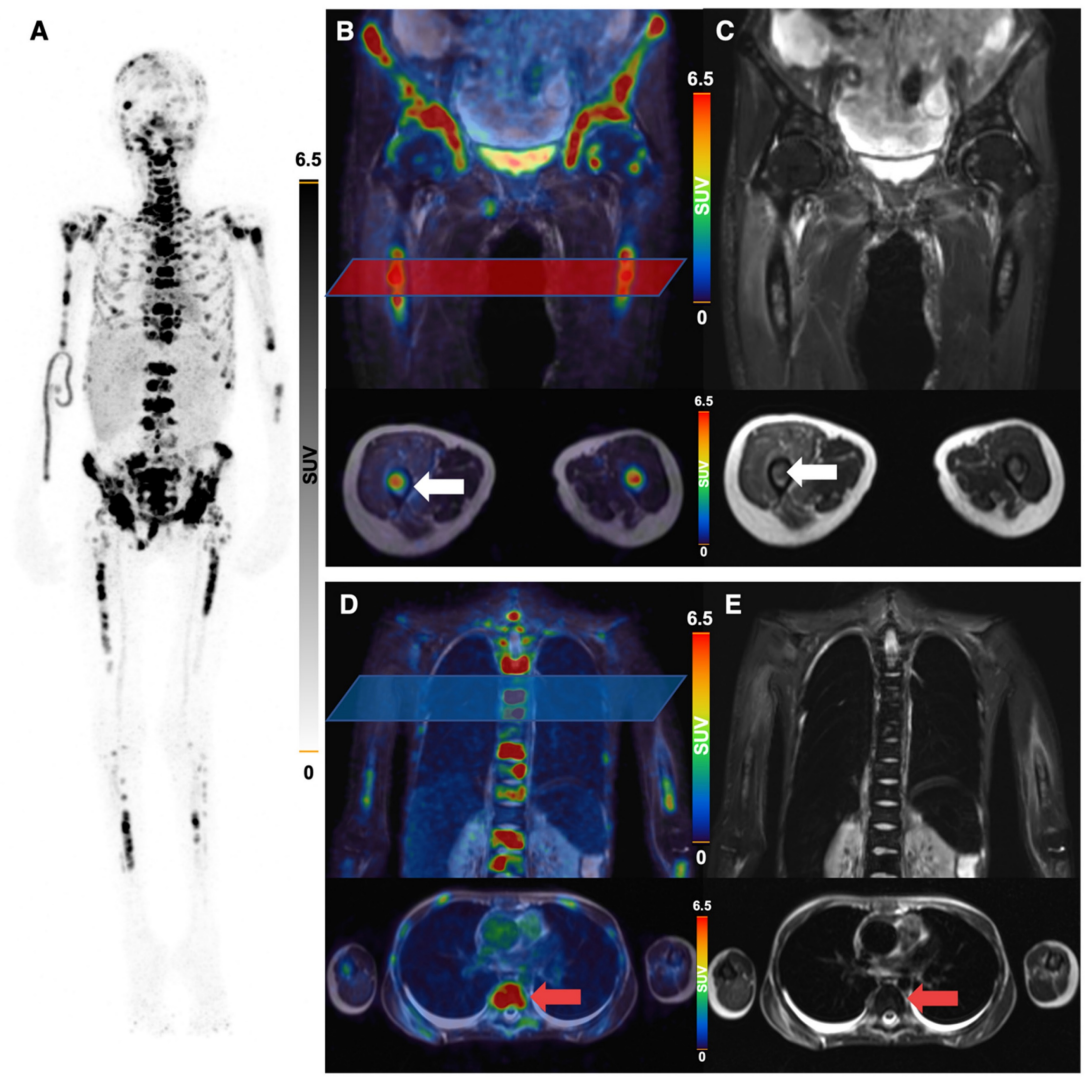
^64^Cu-GD2-PET/MRI of a 20 year old female patient (ID1) with disseminated metastatic neuroblastoma and intense GD2 expression of bone lesions. Maximum intensity projection of PET images on day 1 (A), coronal (upper row) and transaxial (lower row) representation of fused PET/MRI (B, D) and MRI (coronal T2 STIR and transaxial T2 HASTE sequence) (C, E) are shown. The transaxial images correspond to the area of the colored box in the coronal fused images. Multiple ^64^Cu-GD2 positive bone metastases are depicted in the skull, spine, arms and legs with a very high SUVmax up to 30.2 (A). ^64^Cu-GD2 positive metastases are demonstrated in both proximal femora (white arrows; B, C). ^64^Cu-GD2 positive bone metastases with no clear signal in MRI (red arrows; D, E) are shown, revealing the complimentary role of ^64^Cu-GD2 PET for tumor detection.
